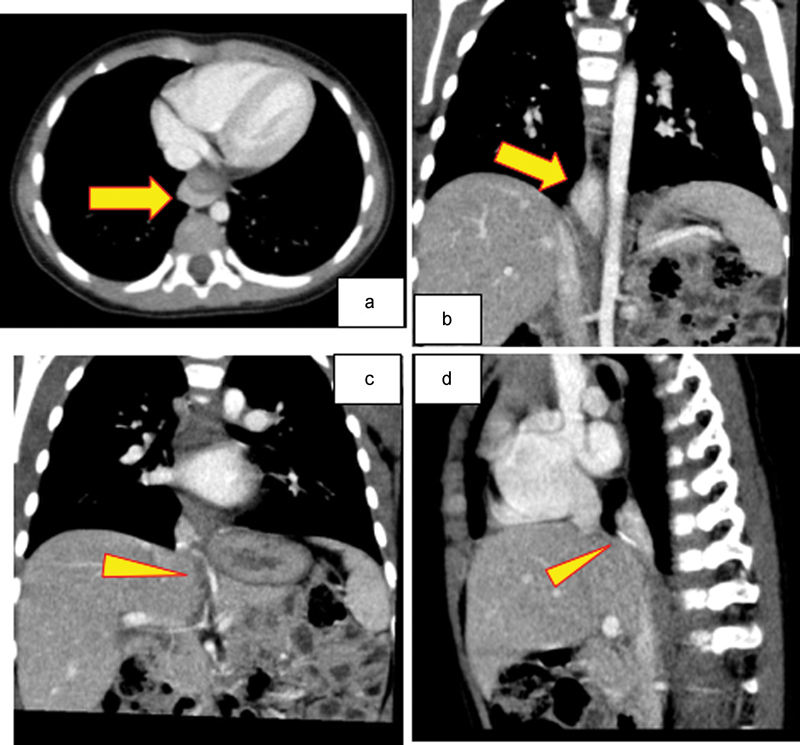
(a, b) Postnatal computed tomography shows two anomalous systemic arteries originating from the left gastric artery and supplying the lung mass. (c, d) All drainage veins in the mass drained to the portal vein.
